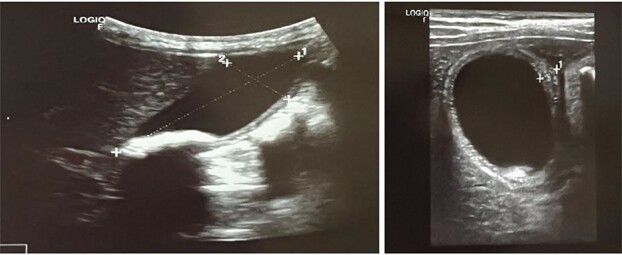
Axial and transverse ultrasonography view, sure wall thickness, and pericholecystic oedema.
